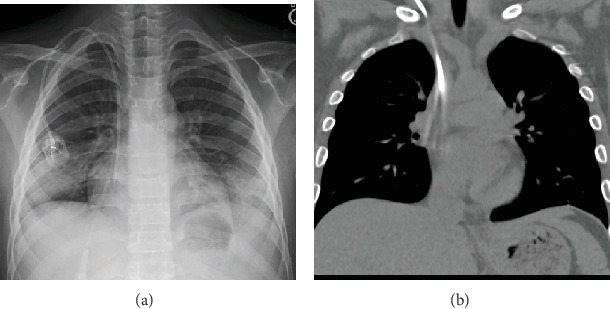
PA chest radiograph (a) demonstrates the placement of a right-sided chest port, with the catheter tip near the cavoatrial junction. Coronal CT (b) done shortly after demonstrates the same catheter, with some motion artifact.
